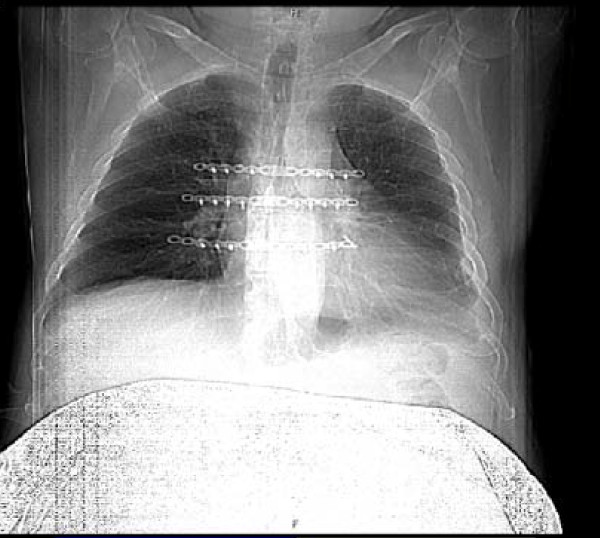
Post-operative Chest X-ray following sternal plates' fixation. It demonstrates sternal union.
